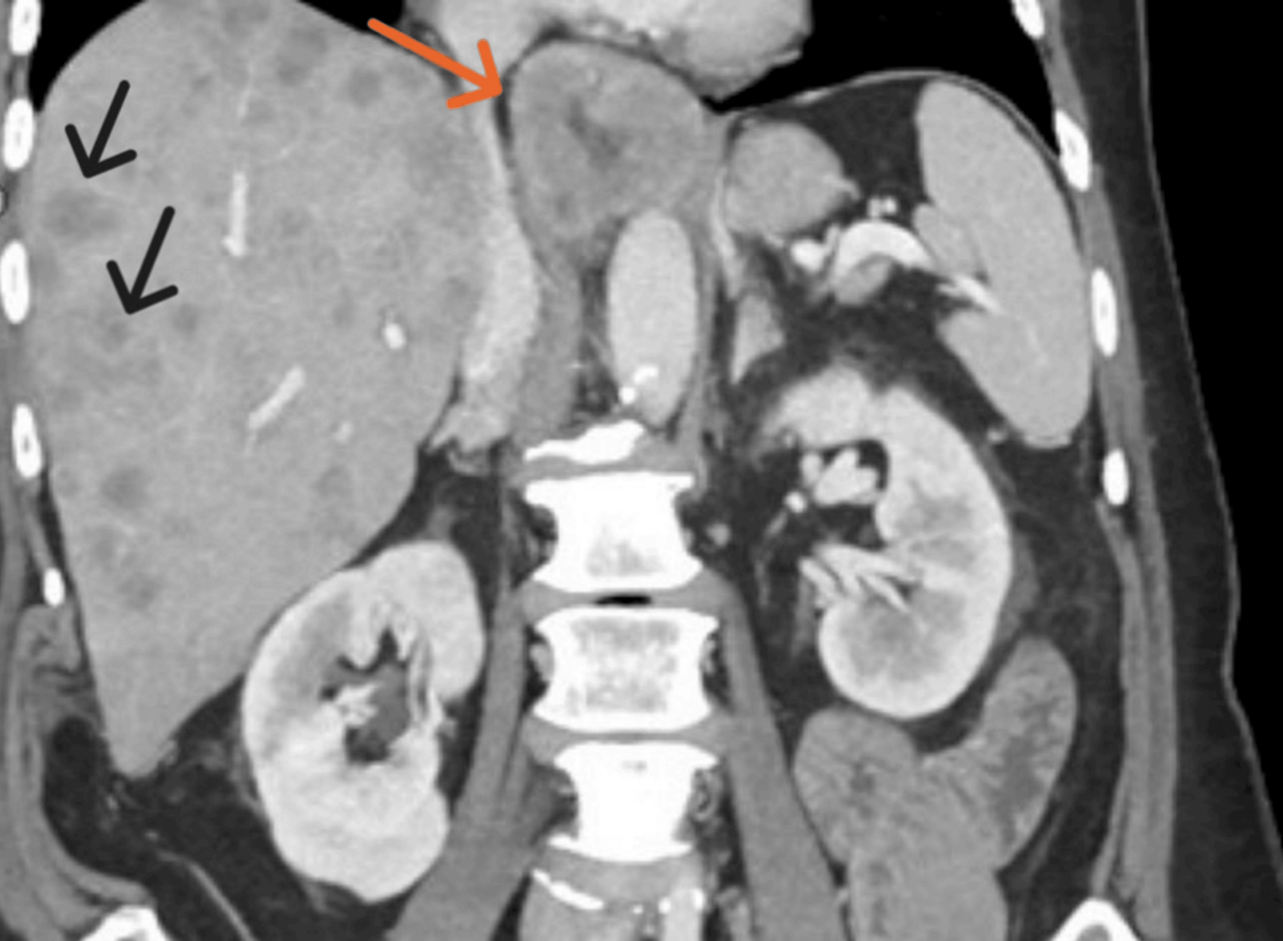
CT of the abdomen, pelvis, and chest Analysis reveals mass-like wall thickening of the distal esophagus (orange arrow), suspected of neoplasm/malignancy. Innumerable hepatic masses (black arrows), suspected of metastases. Gastrohepatic ligament lymphadenopathy, suspected of metastases (not shown). A few pulmonary micronodules up to 3 mm (not shown).
